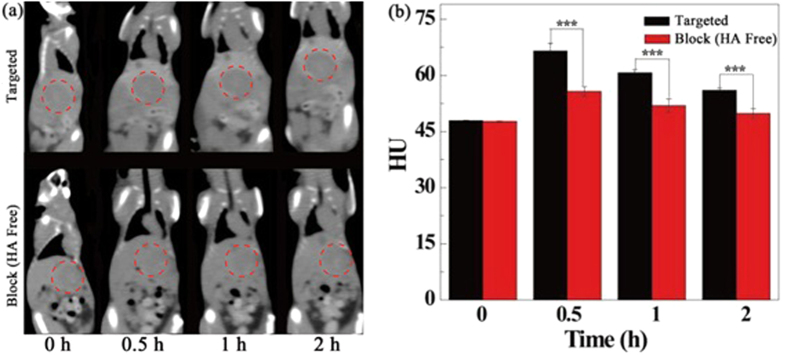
In vivo* CT images of orthotopic liver tumors at different times after a 0.3-mL intravenous injection of a {(Au^0^)_100_G5.NH_2_-FI-DOTA(Mn)-HA} NP solution (0.3 mL in PBS, [Au] = 120 mM).
